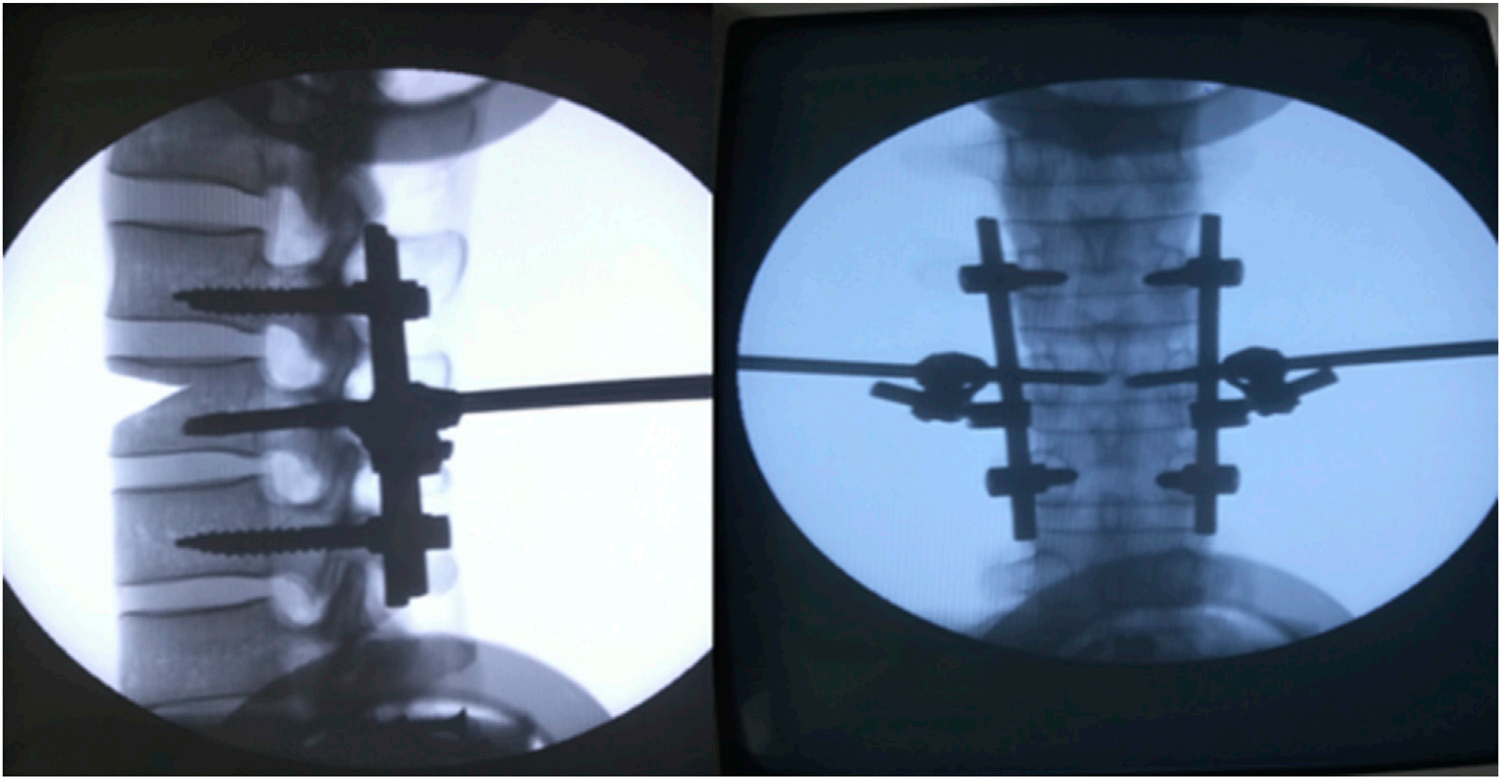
X-rays were used to confirm the success of the internal fixation insertion.
